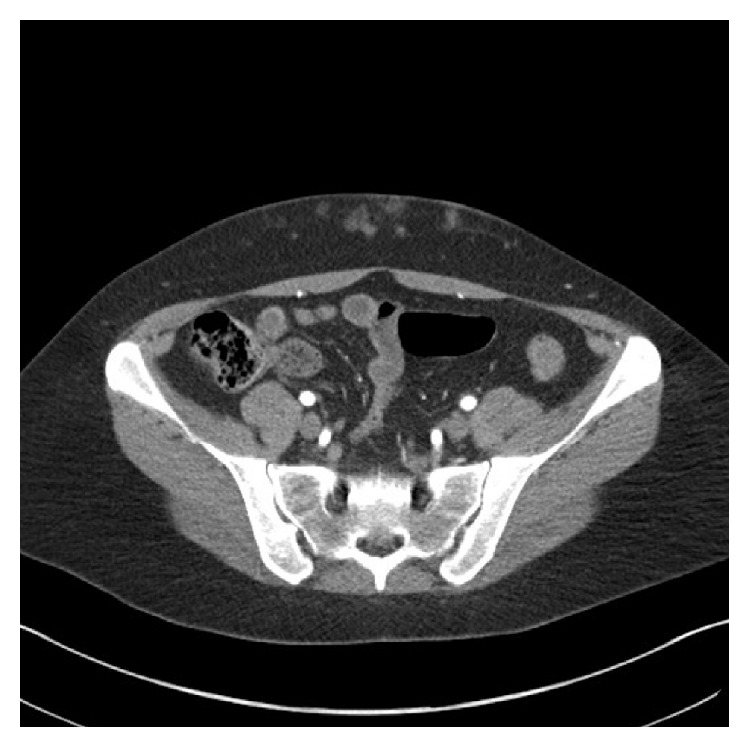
CT angiogram of Patient 3 showing multiple small subcutaneous haemorrhages bilaterally at the sites of heparin injections. DIEP branches appear intact in adjacent fat. The inferior epigastric arteries are seen running posterior to the rectus muscle and sheath.
